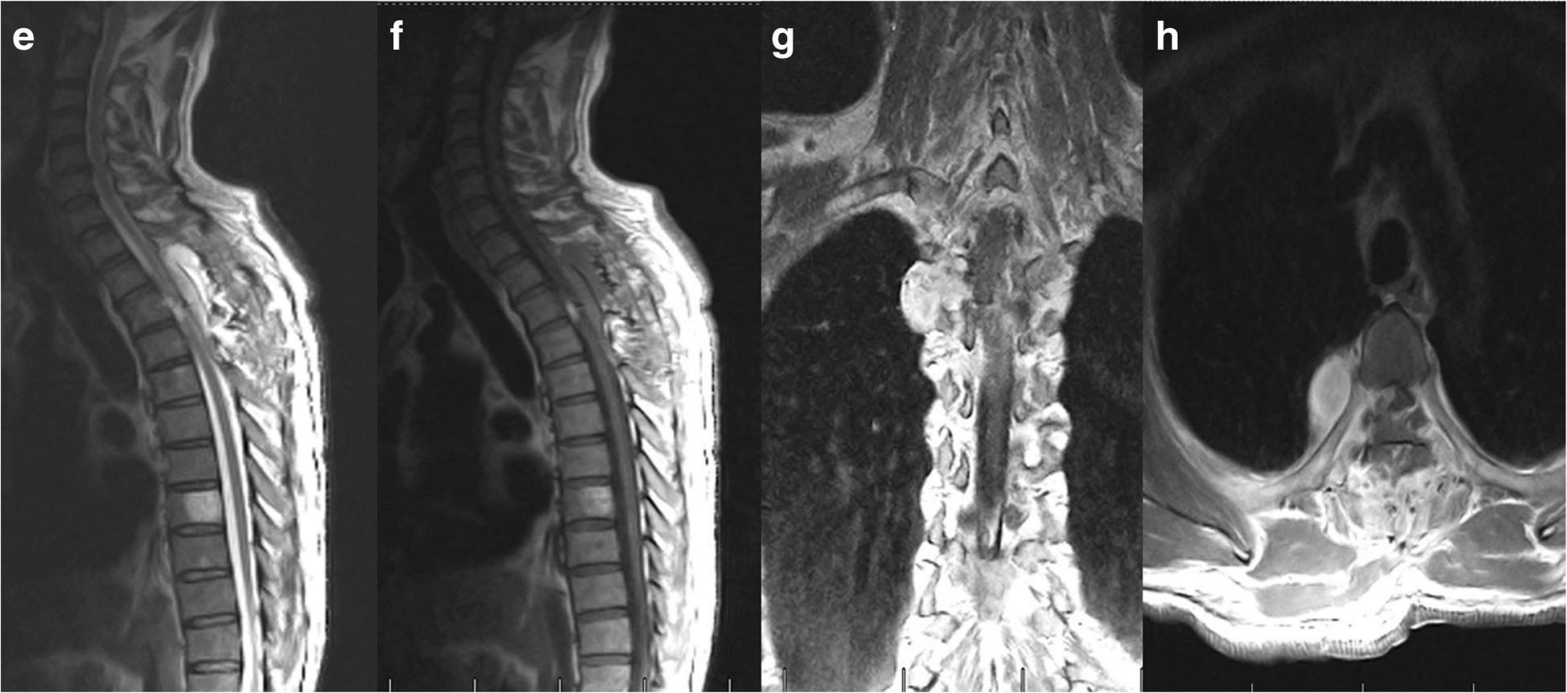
Postoperative sagittal T2-weighted magnetic resonance images (e) and contrast image (f, g and h) shows partial resection (the intraspinal part was totally removed and the extraforaminal portion was not resected)
